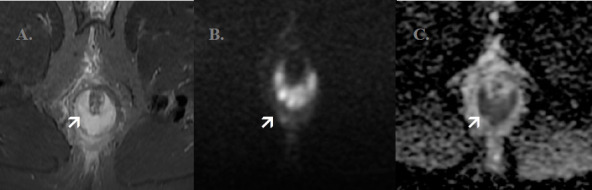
Axial sequence of MRI of a patient with perianal abscess: There is intersphincteric T2 hyperintense collection shown by the arrow in A which has diffusion restriction as shown by the arrow in images B and C
